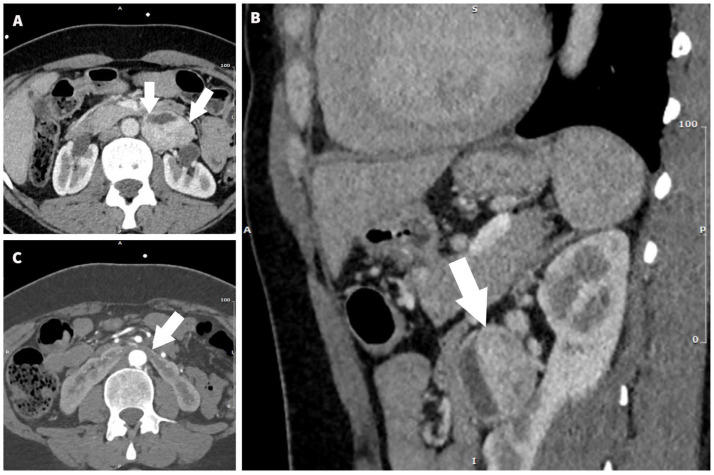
Abdominal CT with contrast shows an inhomogeneous, well-delimited solid mass in the retroperitoneal cavity with areas of necrosis and with strong arterial enhancement (white arrows). (A) Axial section. (B) Sagittal oblique MPR reconstruction. (C) Horseshoe kidney, with symphysis at the level of the lower pole of the right kidney and left kidney.
